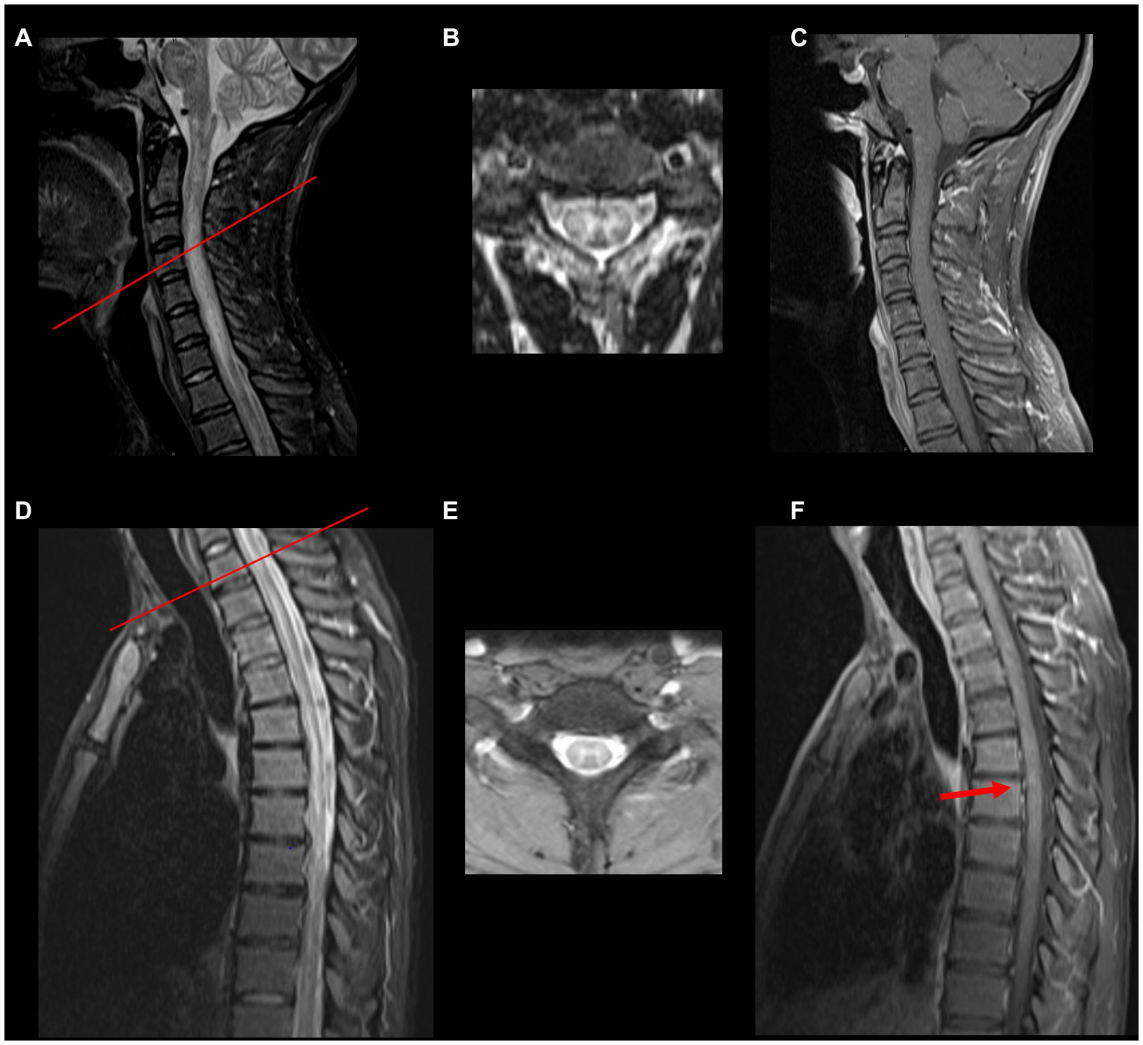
Spinal cord MRI in MOGAD myelitis. (A,D) Abnormal hyperintense T2/FLAIR signal abnormality with cord expansion from the cervicomedullary junction extending to the thoracic spinal cord. (B,E) Show axial T2 images (red bar indicates the spinal cord of the axial section) resembling H sign (grey matter involvement). (C,F) Show post gadolinium T1 sequences, respectively. No enhancement is noted in the cervical spinal cord, but a small focus of enhancement is noted at T9 and T10 (red arrow).
